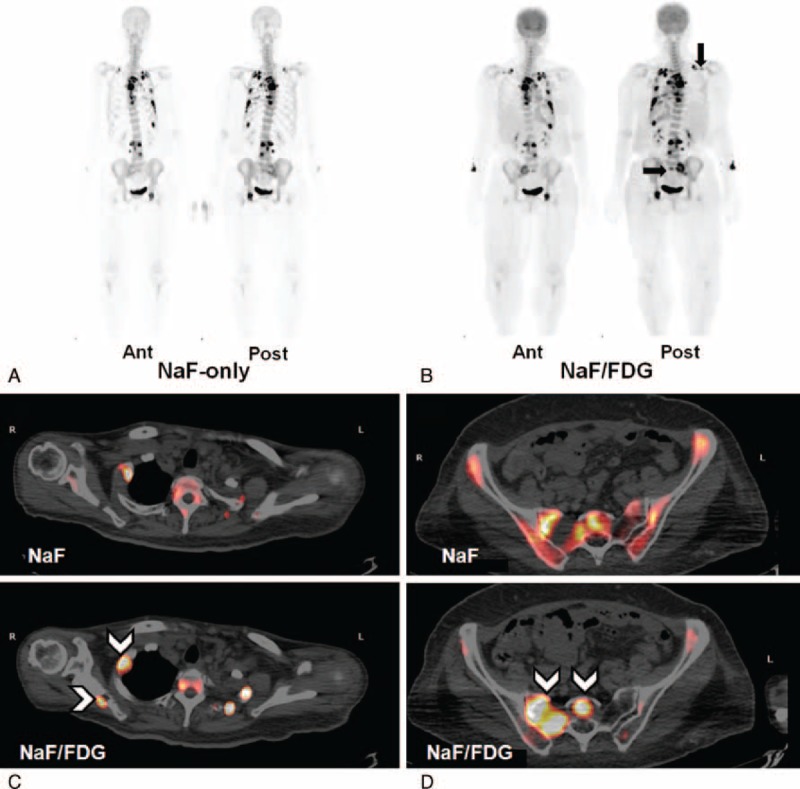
(A) and (B) Maximum intensity projection of F-18 NaF PET/CT and F-18 NaF/FDG PET/CT at anterior and posterior views. The latter radiotracer reveals new foci in the right scapula and sacrum (black arrows), and more extensive uptake in lesions than the former. (C) and (D) Transaxial views of F-18 NaF/FDG PET/CT images show new foci in the right scapula and sacrum, and more extensive radiotracer uptake in the osteolytic portion of the 1st right rib and right sacroiliac region, compared to images using F-18 NaF only (arrow heads). FDG = fluorodeoxyglucose, NaF = sodium fluoride, PET/CT = positron emission tomography/computed tomography.
